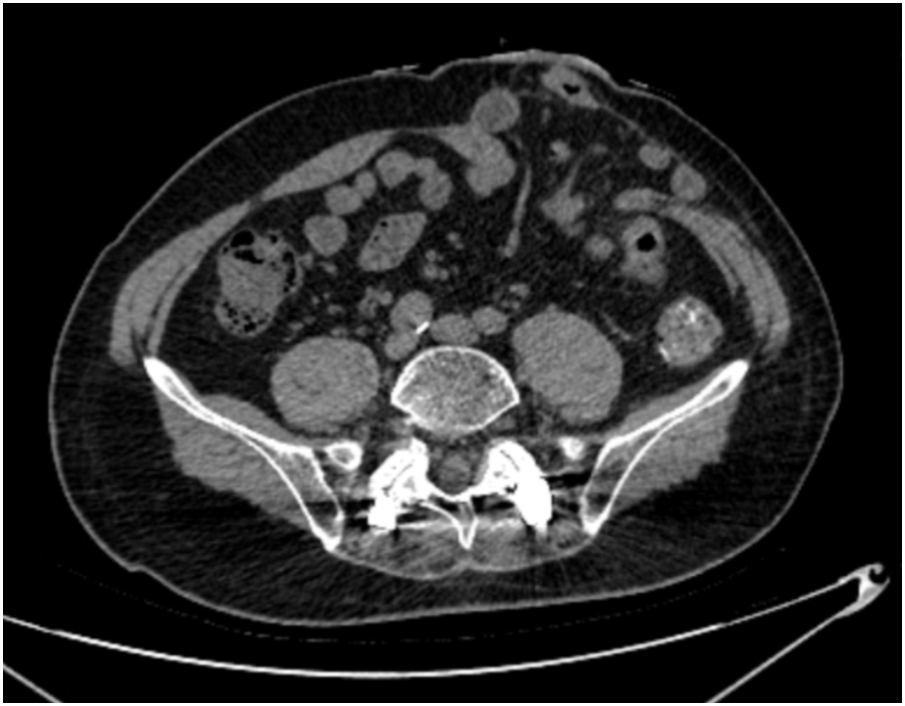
Abdominal CT scan shows herniation of small-bowel loop into PSH.
